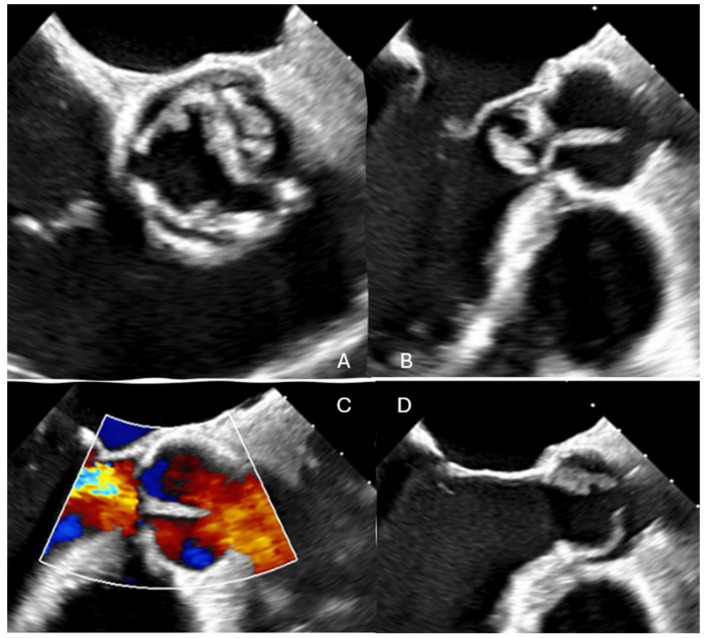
Pre-operative trans-esophageal echocardiography revealed severe aortic regurgitation (C) resulting from extensive destruction of the non-coronary cusp (A,B,D), accompanied by multiple mobile vegetations consistent with active infective endocarditis (A,B,D).
